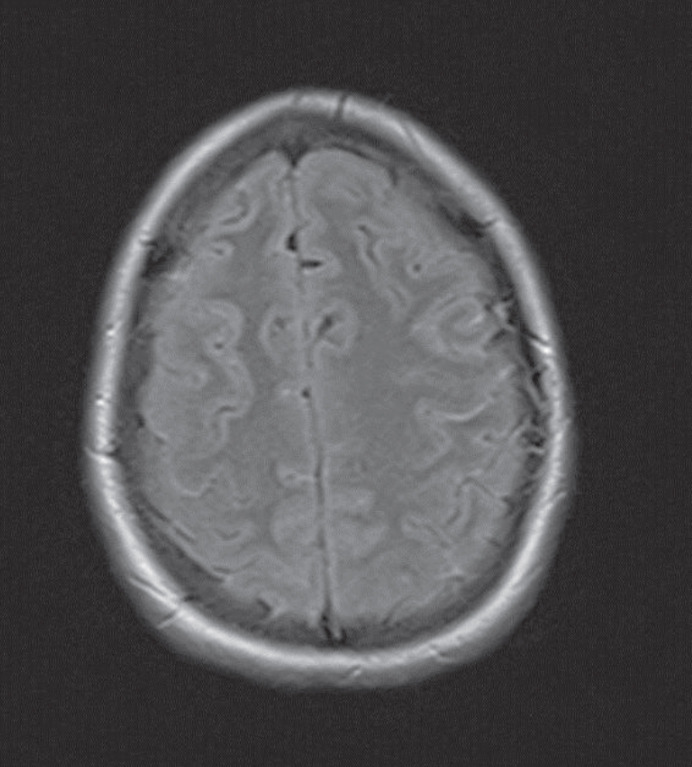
Brain magnetic resonance imaging (MRI) revealed diffuse enhancement after intravenous gadolinium and hyperintensity on fluid-attenuated inversion recovery sequence
